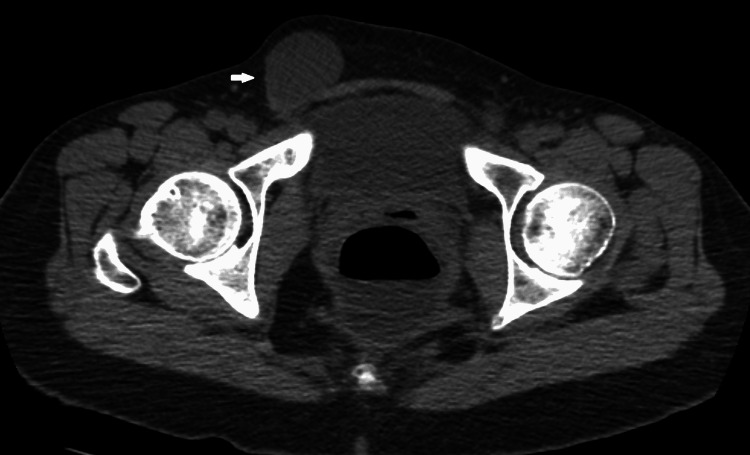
Plain axial CT scan of authors' patient shows comma-shaped hydrocele that contains fluid attenuation (arrow)
